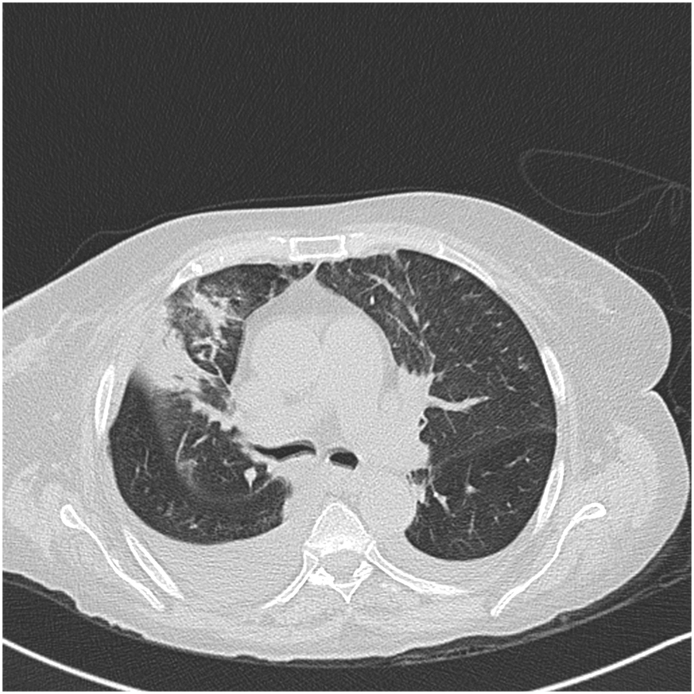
CT-scan showing bilateral distribution of ground glass opacities with consolidation areas in the right lobe.
